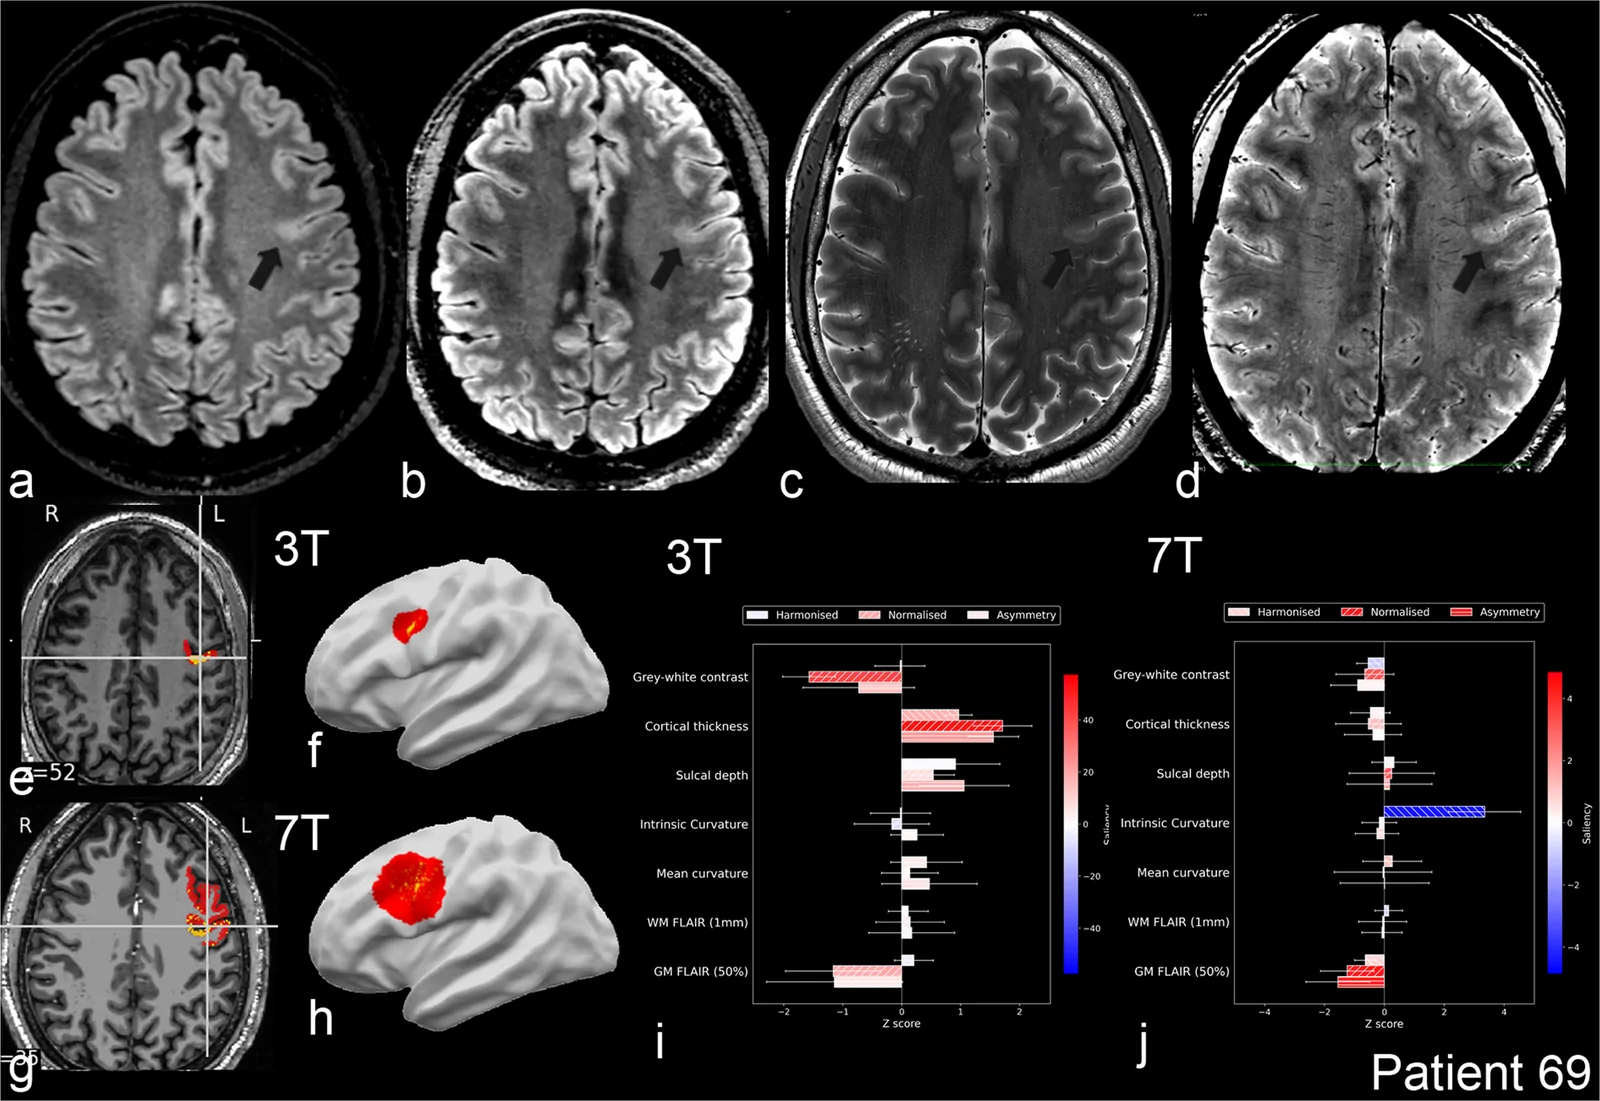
Comparison of 3 T and 7 T classifier results in an 3 T MRI-negative patient (Patient 69). Axial 3 T (a) and 7 T (b) FLAIR MRI, 7 T T2w (c) and Susceptibility-Weighted Angiography (SWAN) (d) MRI; axial 3 T T1w MRI (e) and the corresponding cortical surface 3D model (f) with the lesion cluster detected by the 3 T classifier (red-yellow overlay); axial 7 T T1w MRI (g) and the corresponding cortical surface 3D model (h) with the lesion cluster detected by the 7 T classifier (red-yellow overlay); 3 T (i) and 7 T (j) feature profiles of the clusters generated by the 3T- and 7T-based classifiers. Both the 3 T and 7 T classifiers identified a lesion cluster in the left frontal cortex (e-h), characterized by altered grey-white matter contrast and grey-matter FLAIR signal (i and j). The 3 T classifier revealed also a significant cortical thickening (i) not detected by the 7 T classifier (j)
